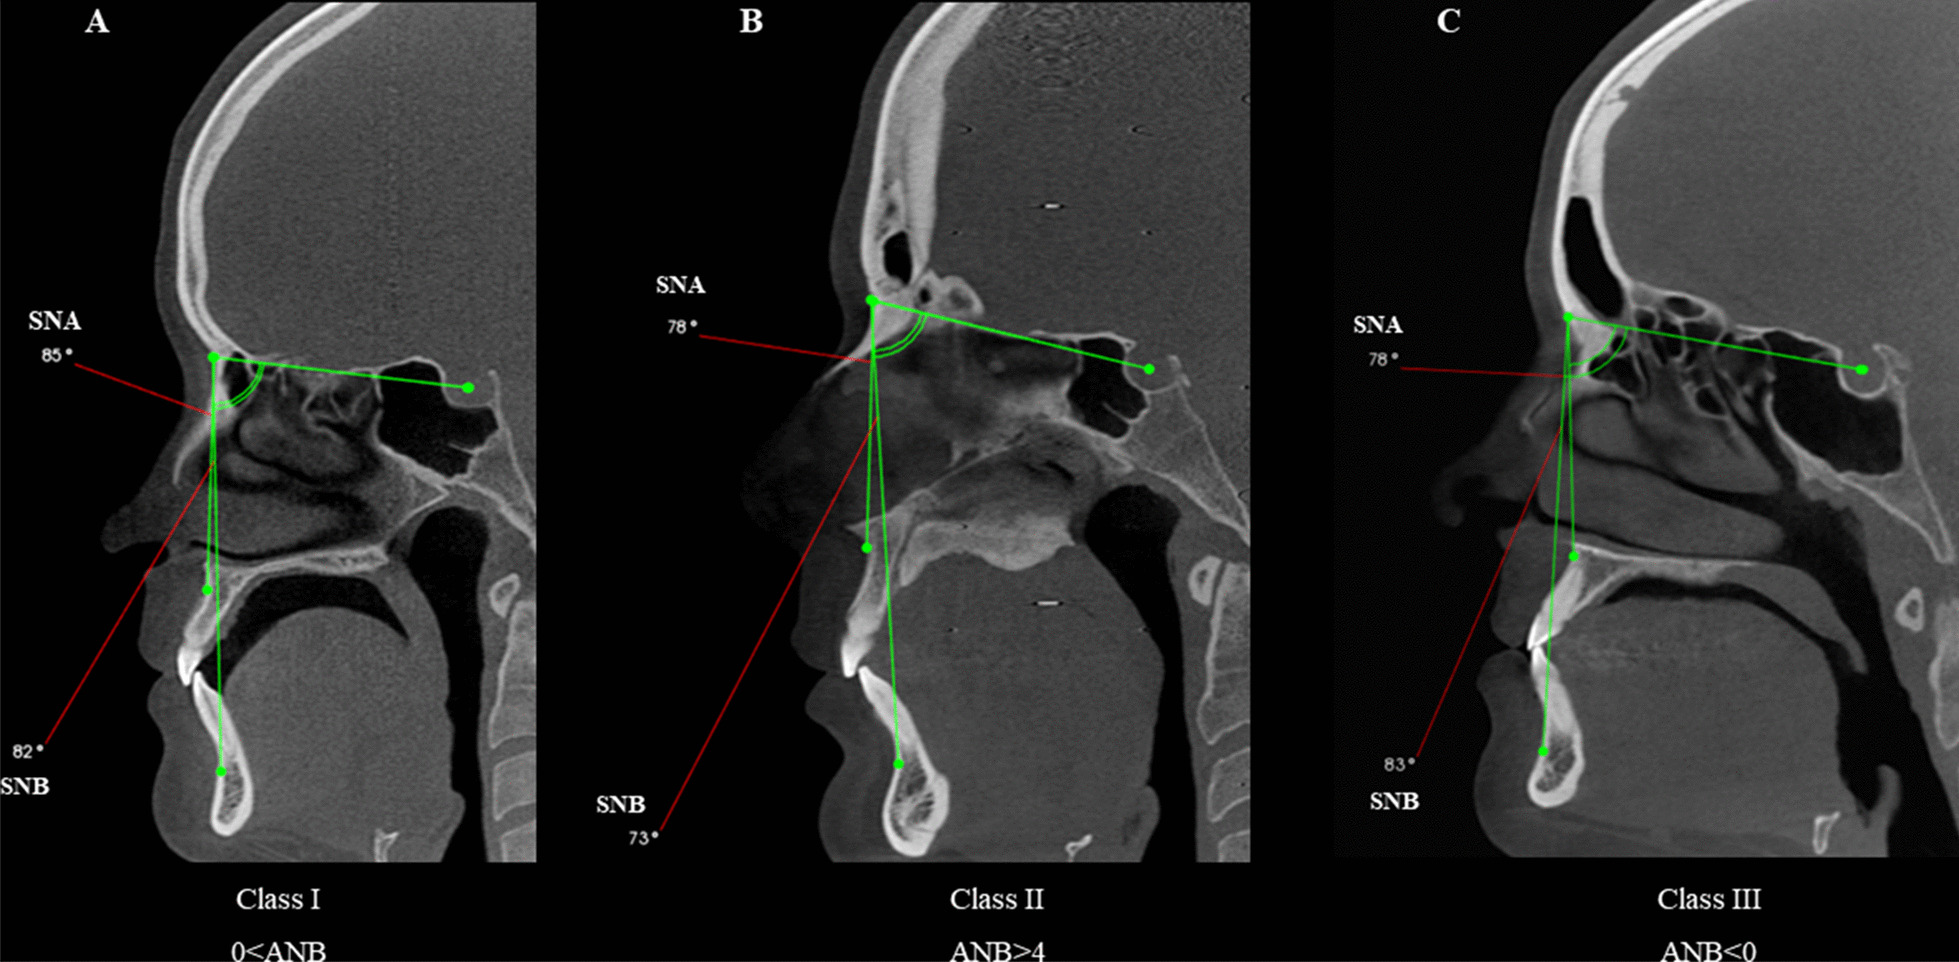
Sagittal reconstructions of cone-beam computed tomography demonstrating patients’ classification according to skeletal malocclusion, based on Steiner’s cephalometric standards for the SNA, SNB, and ANB angles. *SNB-SNA* ANB. A Class I: 0 < ANB < 4, B Class II: ANB > 4, C Class III: ANB < 0
